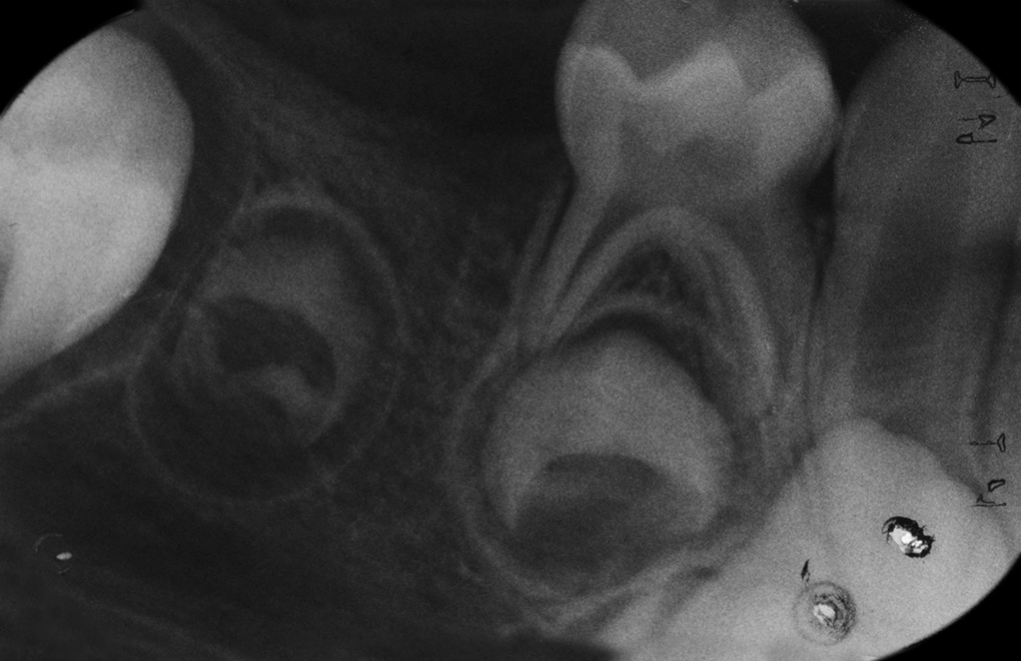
Periapical radiograph, 6 months after the extraction
